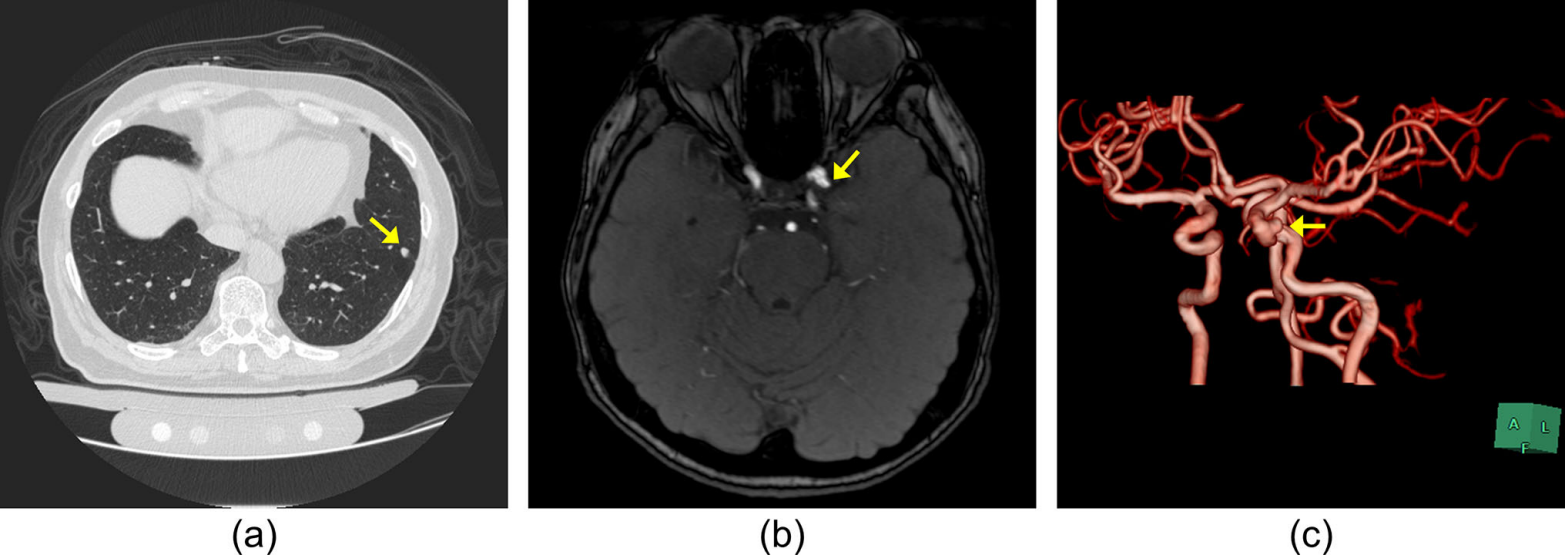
Examples of target lesions included in the test set. a Lung nodule (8.9 mm solid nodule) in chest CT images, b cerebral aneurysm (4.3 mm in left internal carotid artery) in brain MRA images, c pre-rendered whole-brain volume rendering images of the same case as in (b). A yellow arrow indicates the target lesion, but the arrows are not visible during actual reading
